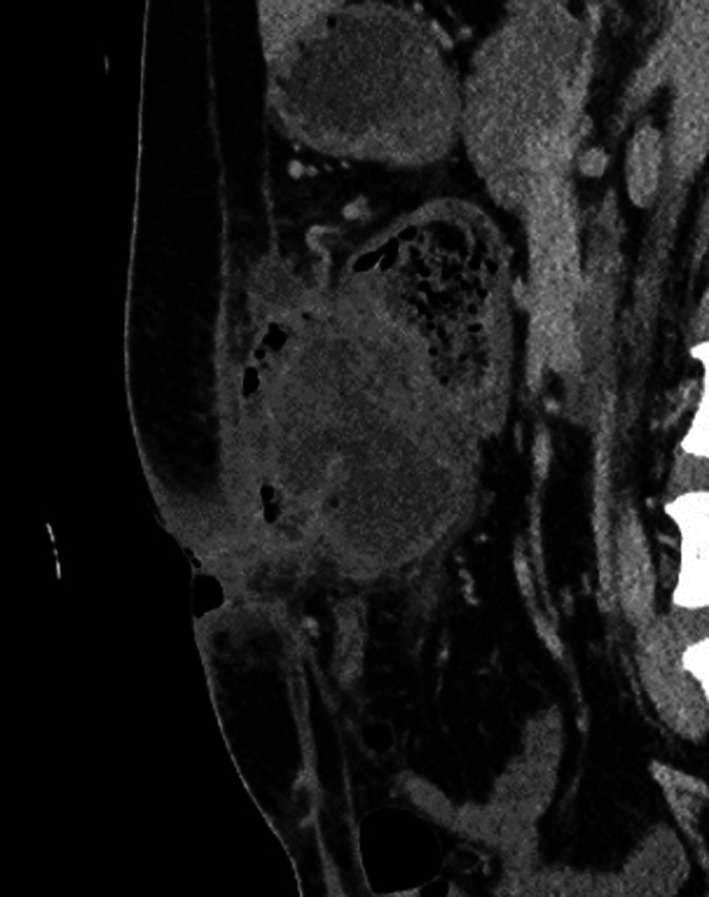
Contrast-enhanced CT scan findings after two courses of FOLFOXIRI. Enhanced CT shows an entero-cutaneous fistula due to tumor penetration of the abdominal wall
